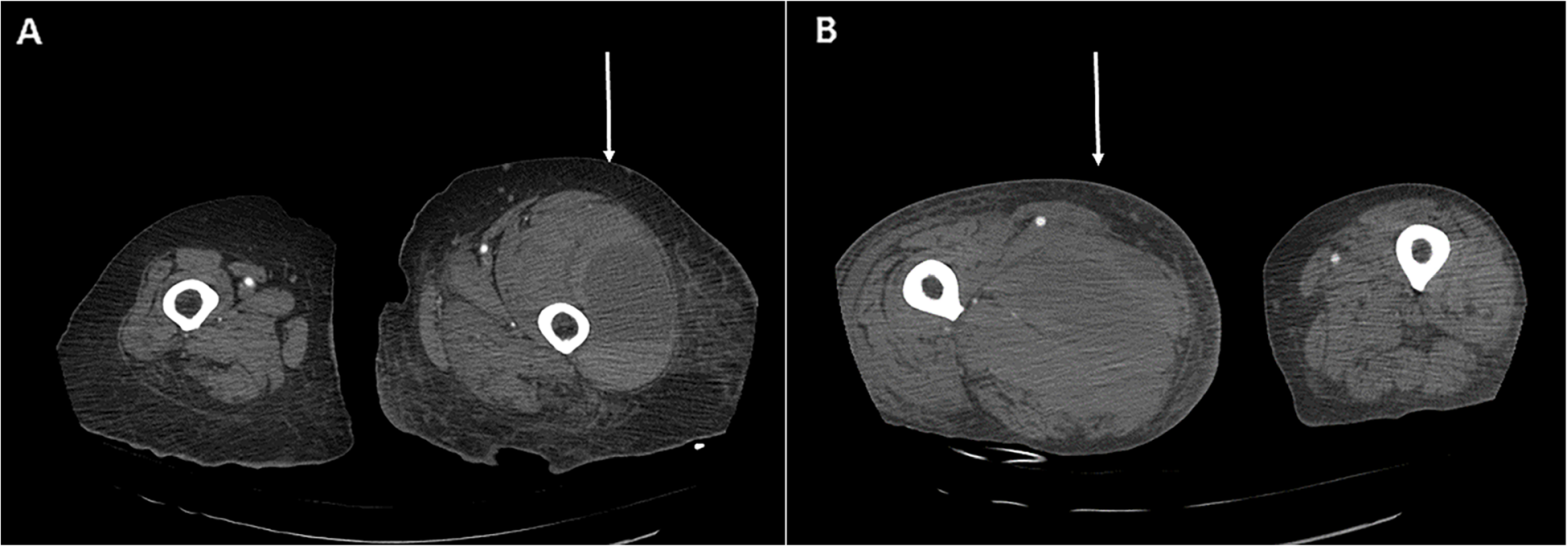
Computed Tomography images of the two patients’ hematomas. This image shows the 11 x 8 x 14 cm hematoma in the Case 1’s left thigh (a) and the 12 x 10 x 25 cm Case 2’s hematoma in the right thigh (b) (white arrows)
